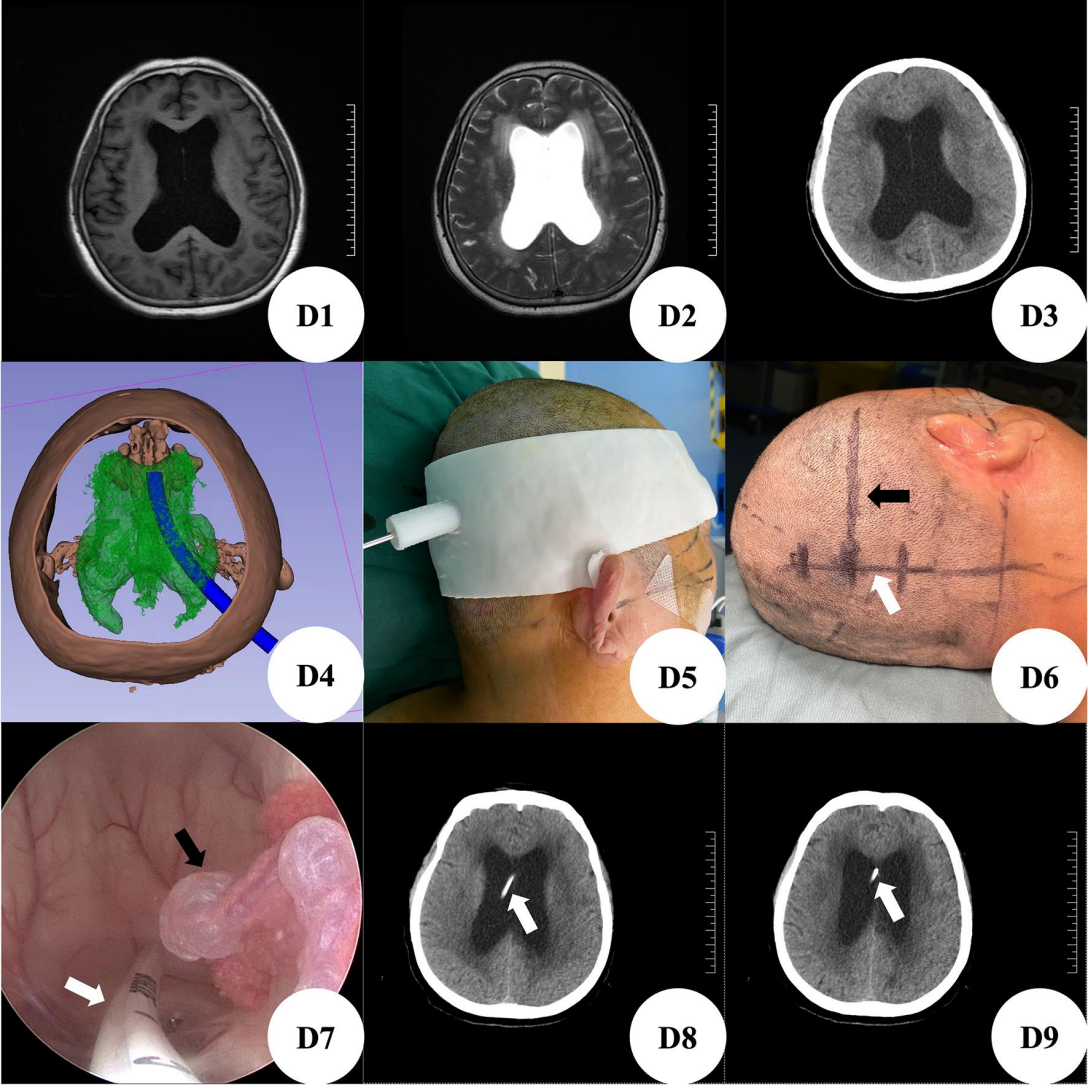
(D1–D2): Preoperative brain MRI showed hydrocephalus and interstitial edema; D3: Preoperative CT showed hydrocephalus and interstitial edema; (D4): 3D-Slicer reconstructed ventricular system and designed puncture channel; (D5): 3D printing positioning guide; D6: The straight incision (white arrow) is designed according to the positioning guide plate, and the black arrow is the puncture direction; (D7): Choroid plexus and ventricular end of shunt tube from the perspective of transcranial neuroendoscopic; (D8–D9): Postoperative brain CT showed that the shunt tube was located in the frontal horn of lateral ventricle.
